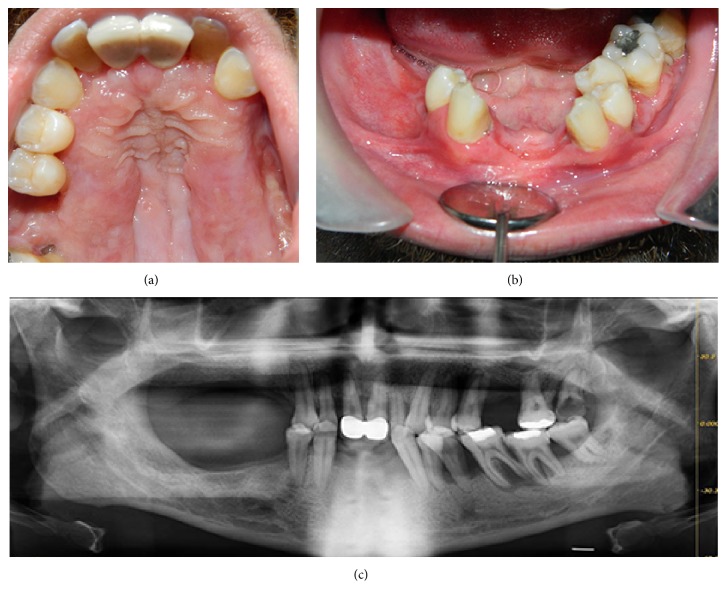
(a) Intraoral view of the patient 12 months after initiation of chemotherapy. Significant regression of gingival lesions is observed on the palatal surface of the maxilla. (b) Intraoral view of the patient 12 months after initiation of chemotherapy. Significant regression of gingival lesions is observed in the mandible. (c) Panoramic radiograph of the patient 12 months after initiation of chemotherapy. Periradicular bone levels of the remaining teeth are maintained except for mandibular left premolar and molar teeth, around which minimal progression of the alveolar bone loss is observed.
